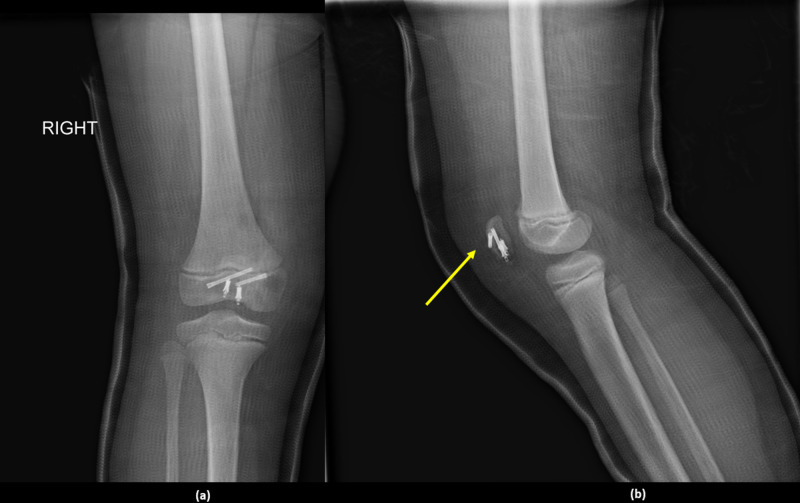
Postoperative radiographs: (a) anteroposterior view and (b) lateral view
